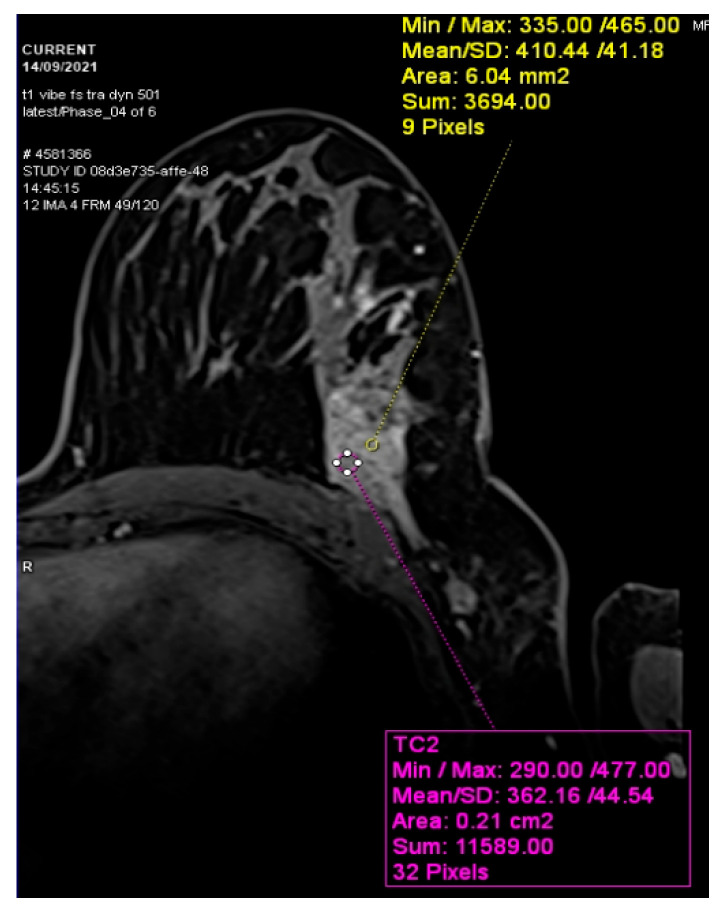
Left breast tumor MRI aspect (institutional patient data).
